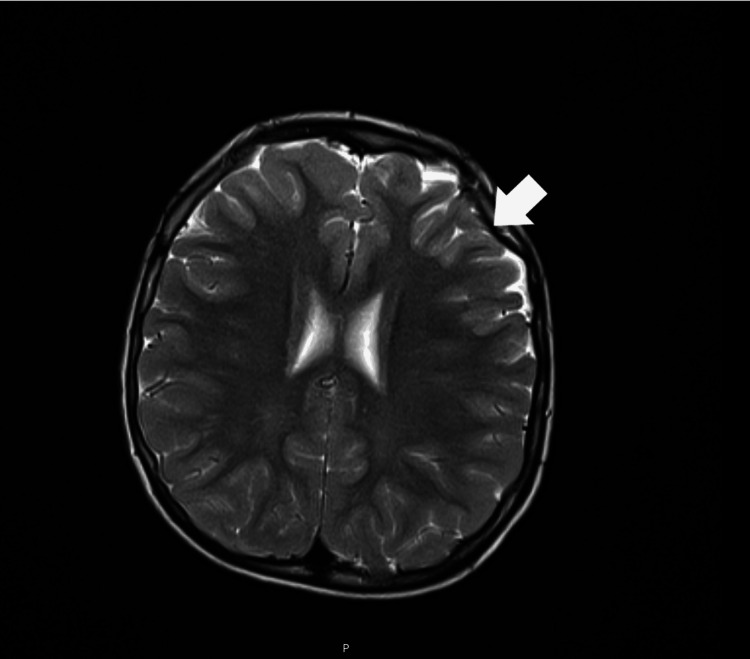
Patient 2's brain MRI, performed at the age of seven. Seen is a slight frontal bilateral atrophy, more evident on the left (arrow).
